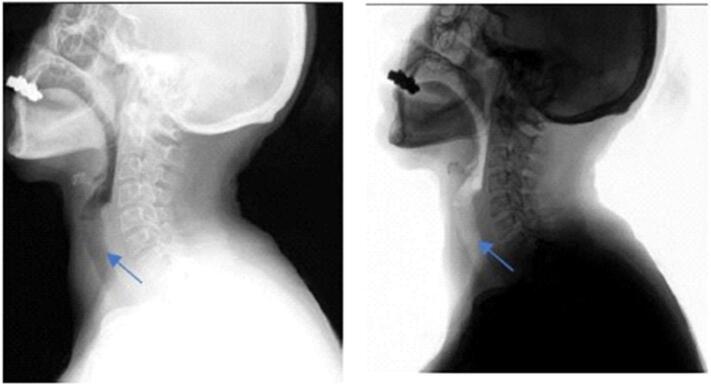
Lateral neck radiograph showed a linear radiopacity in the region of cricopharynx suggestive of a foreign body; Normal thickness of retropharyngeal and retrotracheal space.
